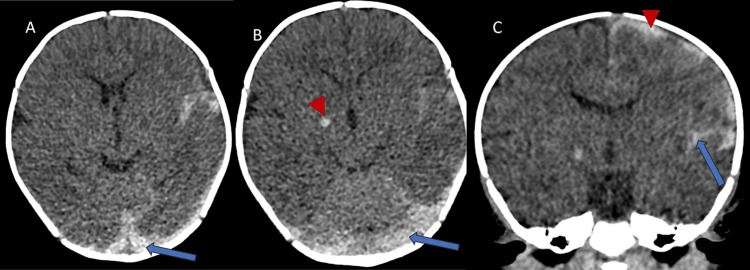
Unenhanced CT scan of head: axial images show bilateral subdural hemorrhages (arrow in A and B), right basal ganglia hemorrhage (arrowhead in B), subarachnoid hemorrhage (arrow in C) and subpial hemorrhage (arrowhead in C). There is diffuse cerebral edema with loss of gray-white differentiation
